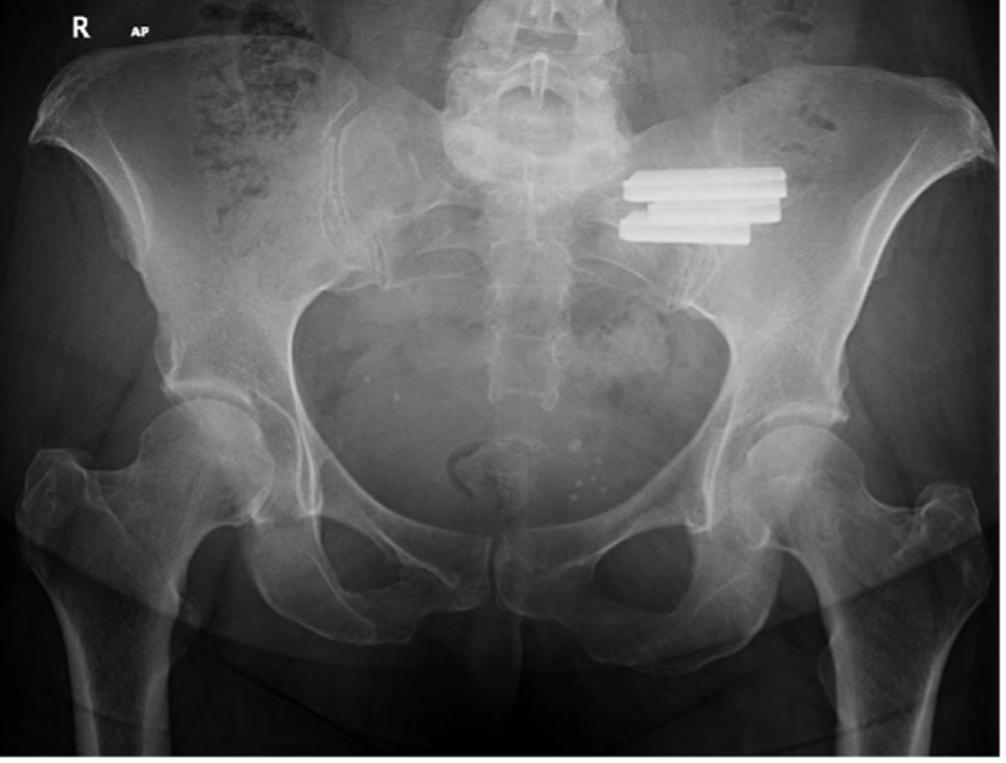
Ap X-ray of a pelvis, showing 1 year follow of left sacroiliac fusion, note absence of lysis or displacement, this was considered a radiological success.
